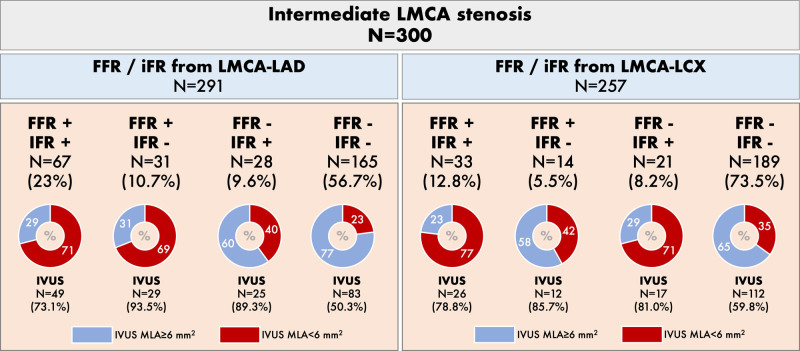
Patient classification according to the concordance between fractional flow reserve (FFR) and instantaneous wave-free ratio (iFR) as well as intravascular ultrasound (IVUS) significance. FFR and iFR were measured from left anterior descending coronary artery (LAD) in 291 patients and from left circumflex coronary artery (LCX) in 257 patients. There were 3 patients with measurements from LCX but not from the LAD, and 6 patients only with iFR measurement. LMCA indicates left main coronary artery; and MLA, minimal lumen area.
